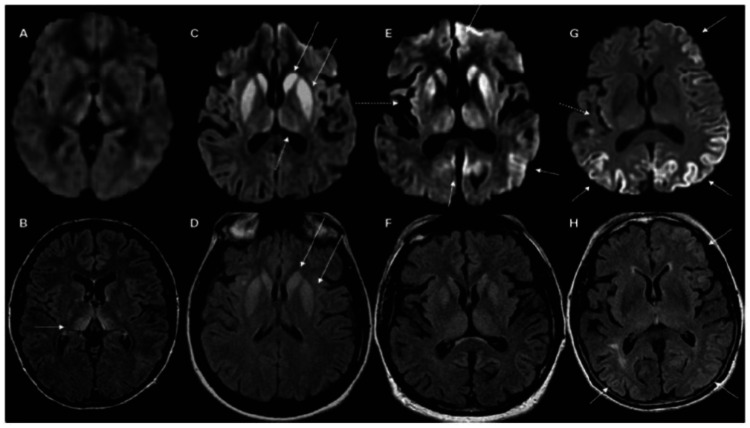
Abnormal MRI findings in sCJD; C, D: Subcortical lesions; E, F: Both cortical and subcortical lesions; G, H: Cortical lesions. A, C, E, and G are DWI images that are more prominent, while B, D, F, and H are FLAIR images. Original figure attributed to Staffaroni AM, Elahi FM, McDermott D, et al.: Neuroimaging in dementia. Semin Neurol. 2017, 37:510-537. 10.1055/s-0037-1608808 and permission was obtained from the original publisher. MRI: magnetic resonance imaging; sCJD: Sporadic Creutzfeldt Jakob Disease; DWI: diffusion-weighted imaging; FLAIR: fluid-attenuated inversion recovery.
